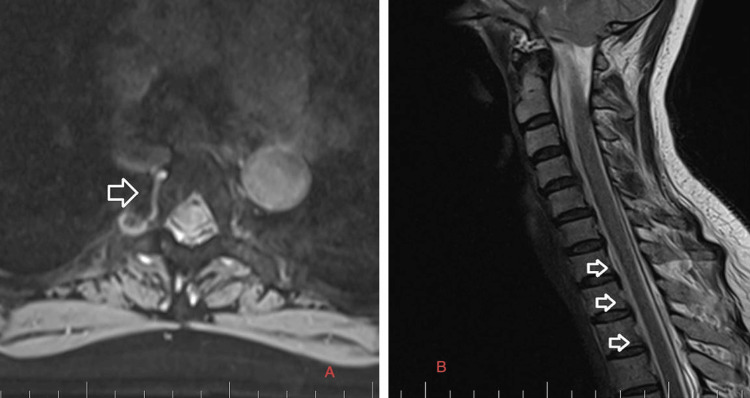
C spine MRI on February 2, 2023. A: Axial T2-weighted images. The white arrow shows the T1 right foraminal cerebrospinal fluid leak. B: Sagittal T2-weighted images. The white arrows show the spinal longitudinal extradural fluid collection type 1b.
